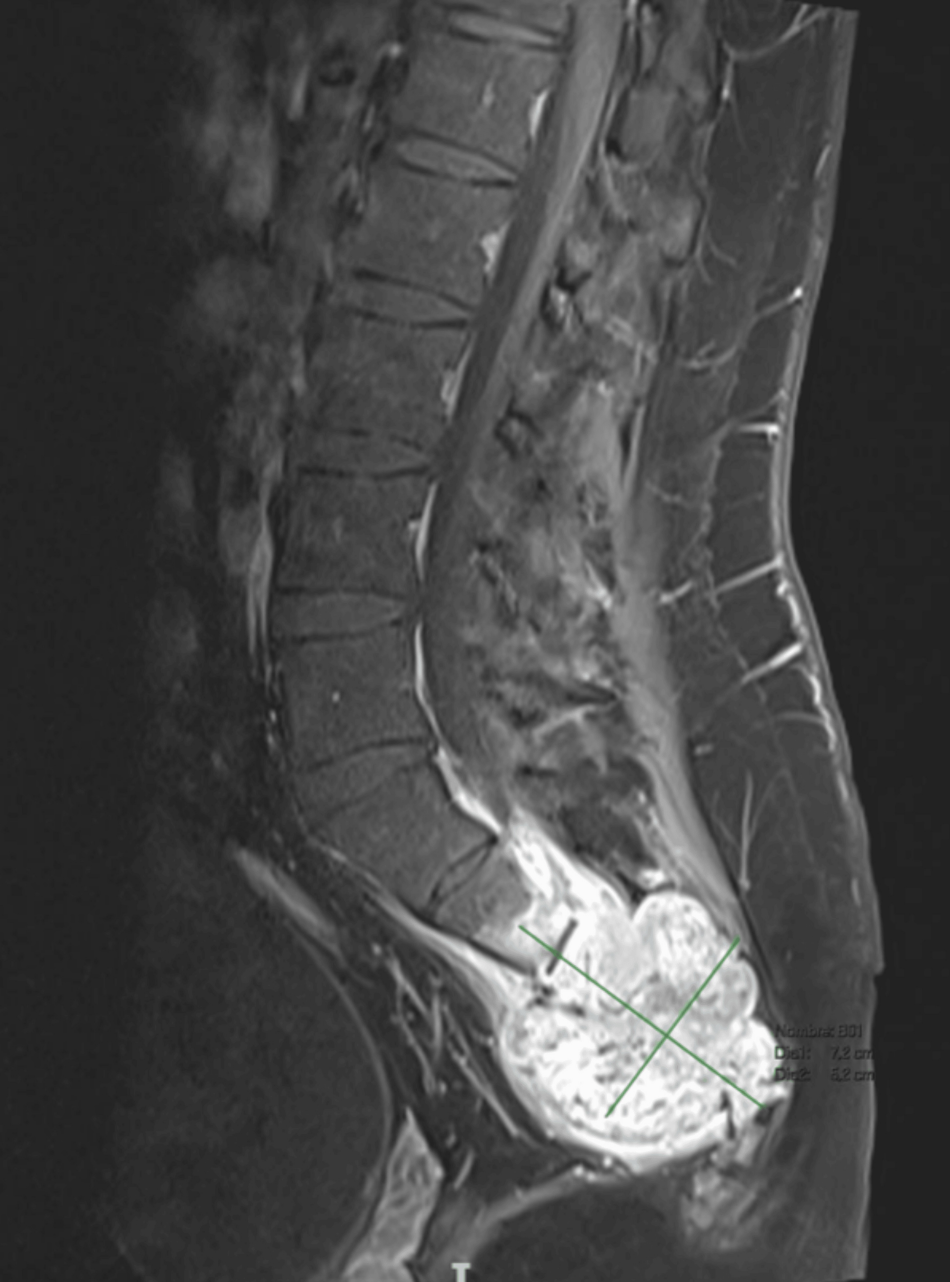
Contrast-enhanced T1-weighted MRI sequence. The sacral mass measured 7.2 cm × 5.2 cm.
